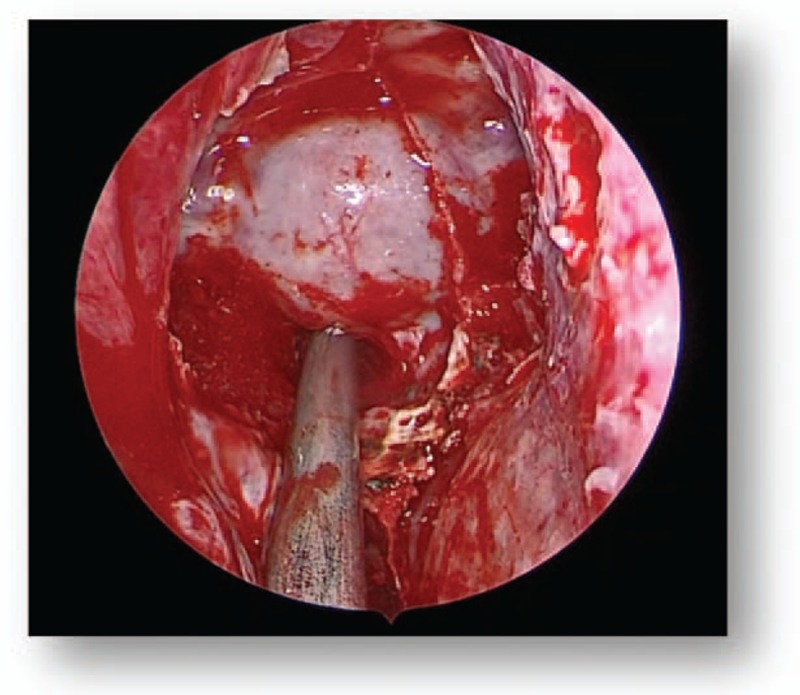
Preoperative MRI (B). MRI = magnetic resonance imaging.
